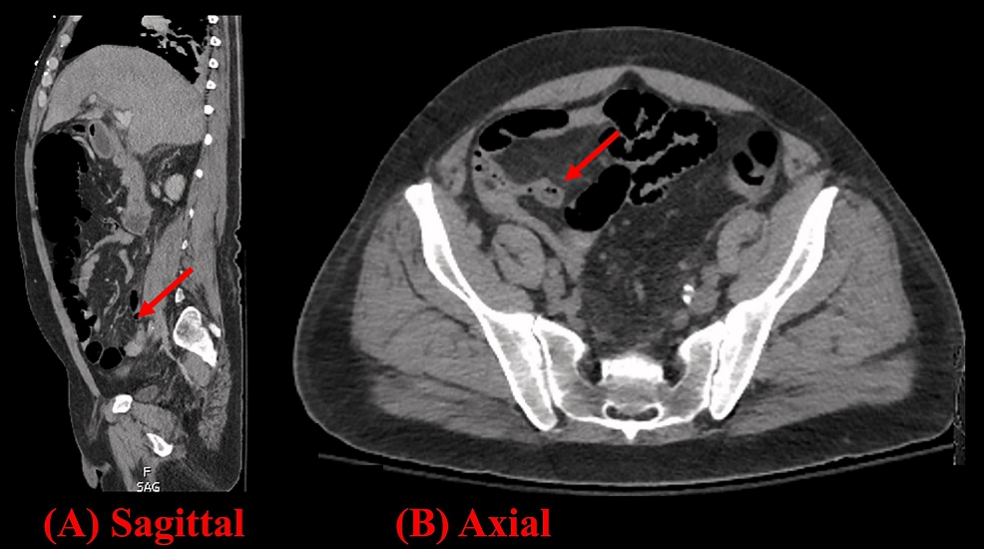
CT abdomen and pelvis coronal (A) and axial (B) images showing a fat-density, ovoid structure (arrow) adjacent to the colon with a thin high-density rim (hyperattenuating ring sign) consistent with epiploic appendagitis.
